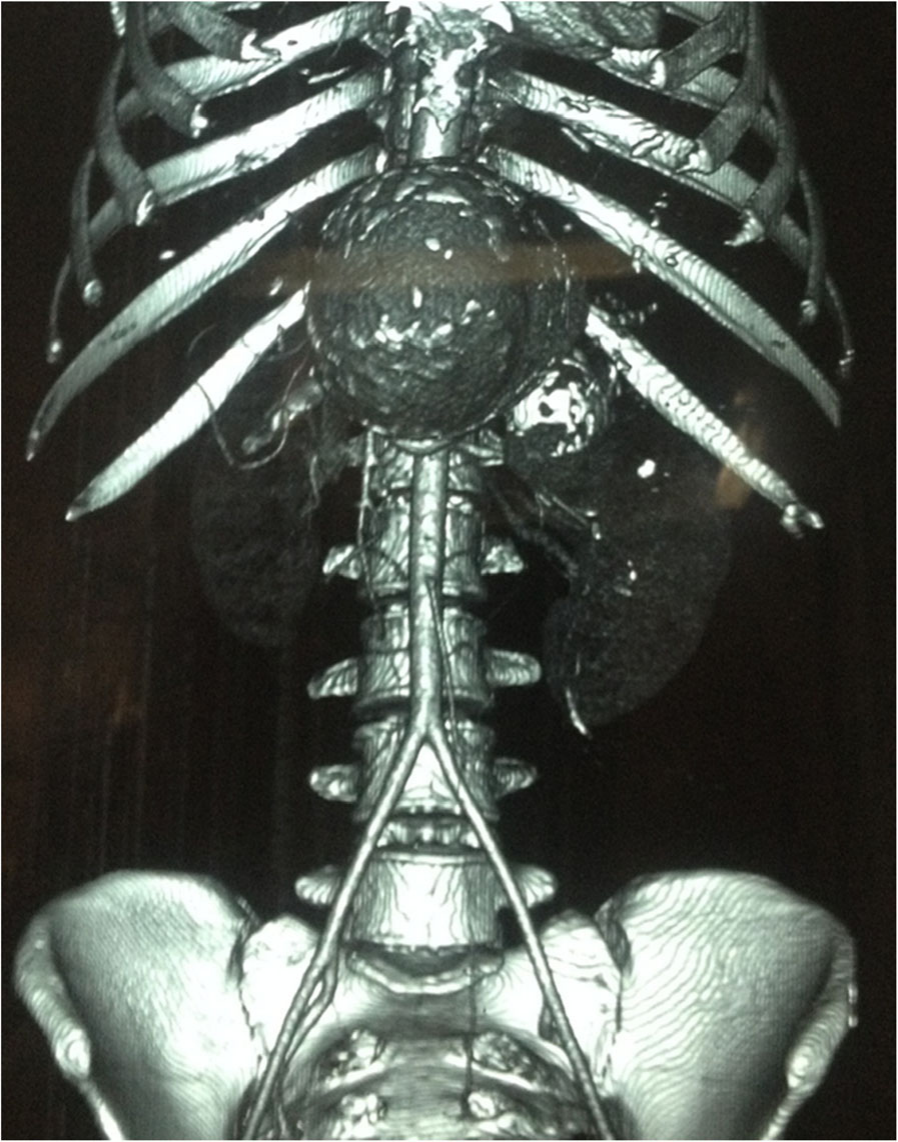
Computed tomography angiogram of our patient showing a giant splenic artery aneurysm. The computed tomography angiogram was taken with permission from our patient
